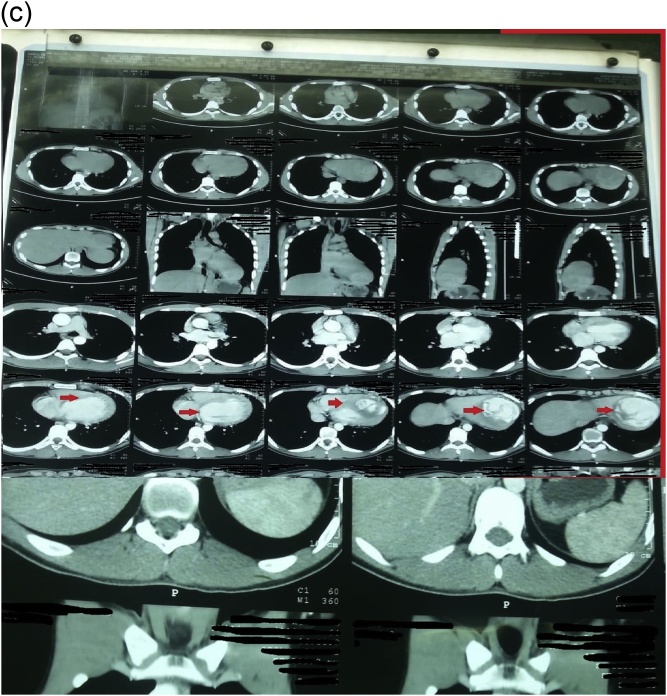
(a) Chest CT scan with hydatid cyst in the left ventricle (2). (b) CT scan of chest show left ventricle hydatid (2). (c) CT scan hydatid cyst in LV with membrane disloged and rupture (2).
